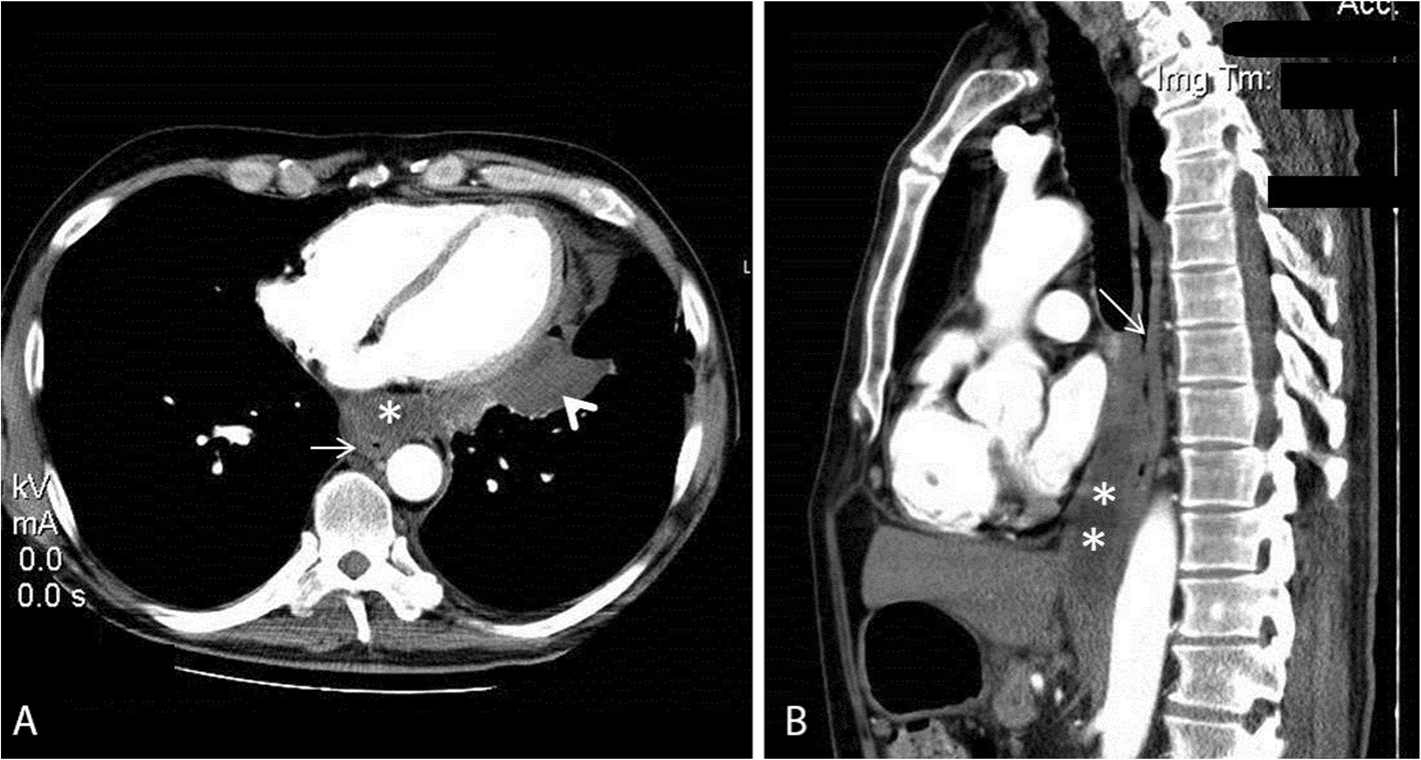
Post-contrast chest computed tomography scan after successful pleural drainage. Panel A: Fluid collection (*) in front of esophagus (arrow) and a small pleural effusion is present (arrow head). Panel B: Sagittal reconstructed images demonstrate a longitudinal fluid collection (* *) in the mediastinum, along the lower esophagus (arrow), measuring 3×8cm. The lesion was compatible with a mediastinum pseudocyst.
